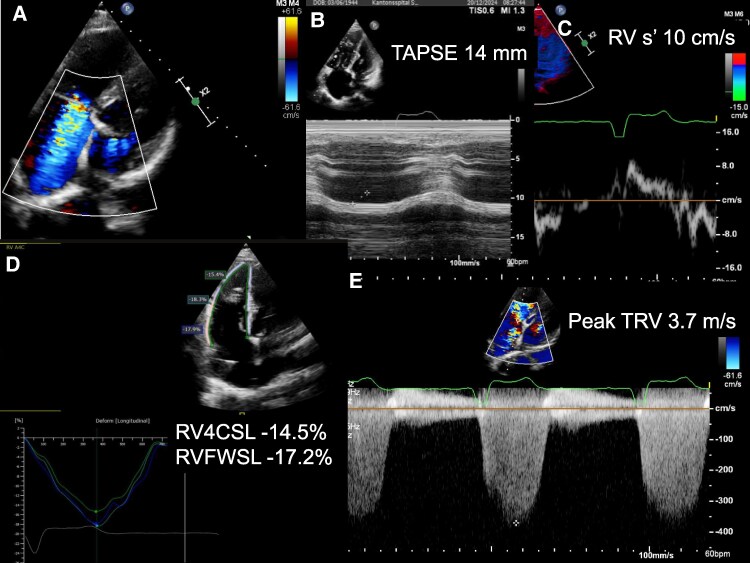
Transthoracic echocardiography. (*A*) Atypical four-chamber view showing severe tricuspid regurgitation. (*B*) M-mode showing reduced tricuspid annular plane systolic excursion (14 mm). (*C*) Pulsed wave tissue Doppler showing borderline right ventricular S’ (10 cm/s). (*D*) Speckle tracking showing reduced right ventricular four-chamber longitudinal strain and reduced right ventricular free wall longitudinal strain. (*E*) Continuous wave Doppler showing increased peak tricuspid regurgitant velocity (3.7 m/s) suggestive of a high probability of pulmonary hypertension.
